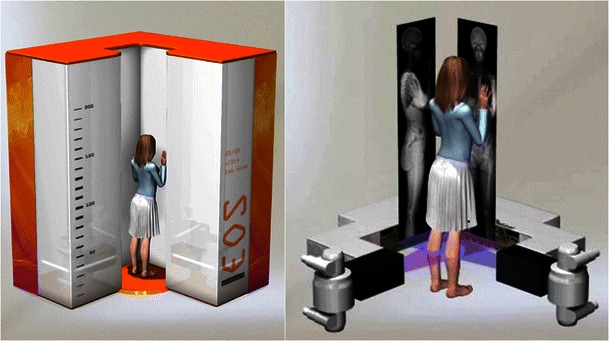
Imaging technique of the EOS 2D system. The gantry is composed of two sets of X-ray tubes and detectors positioned orthogonally and supported by a mobile arm. This arm moves vertically while the patient is positioned upright at the intersection of the two X-ray fan-beams. A single scan can produce both AP and lateral radiographs of the spine, the lower limbs or the whole skeleton
